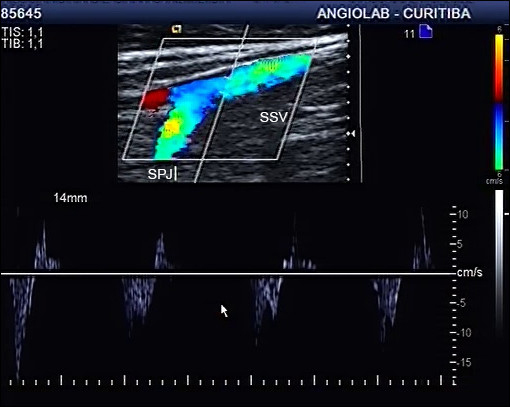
Ultrasound image of the small saphenous vein (same case as in Figure 4) without reflux, demonstrating that reflux in the Giacomini vein is drained via the saphenopopliteal junction. SSV = Small saphenous vein; SPJ = saphenopopliteal junction.
